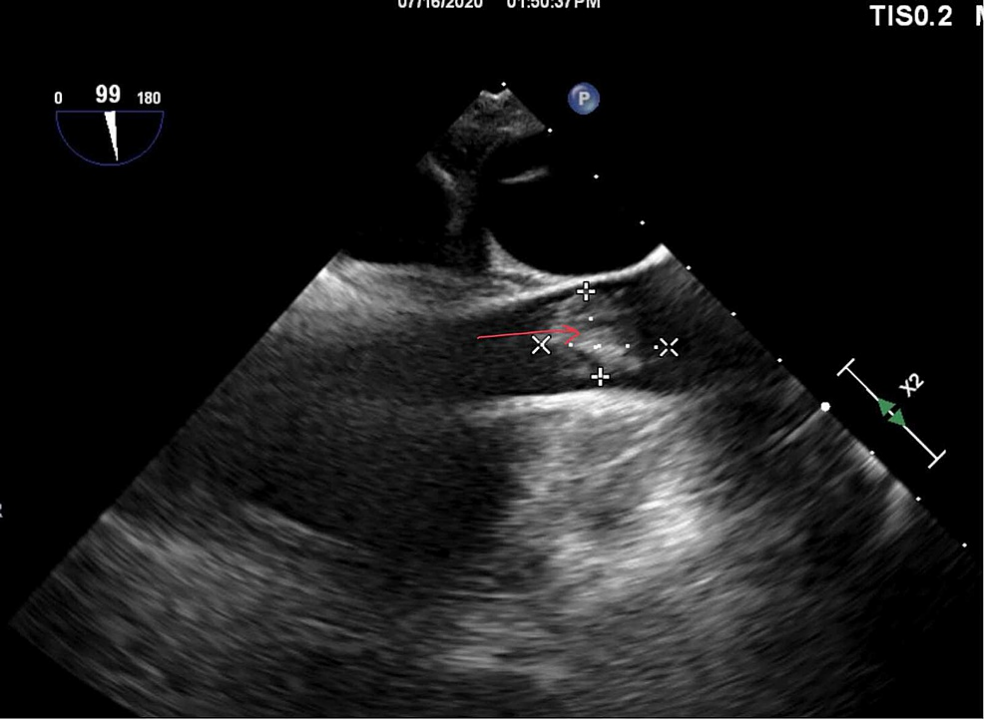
TEE showing greater than 70% debulking of the superior vena cava vegetation TEE: transesophageal echocardiography
